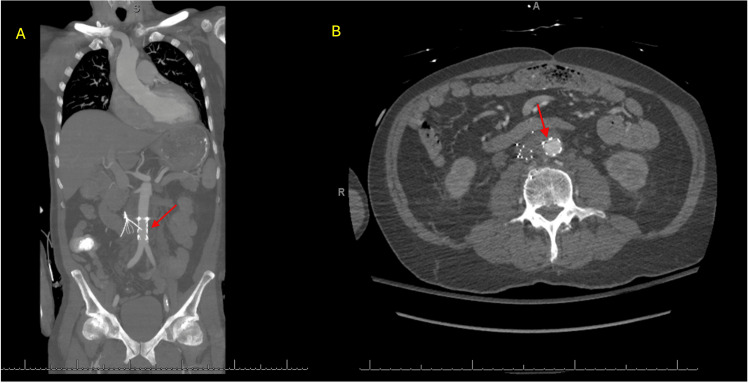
CT demonstrating endovascular aortic repair (EVAR) with resolution of active extravasation A) Coronal view demonstrating the Gore extender graft (red arrow) with resolution of active extravasation. B) Axial view demonstrating the Gore extender graft (red arrow) with resolution of active extravasation.
